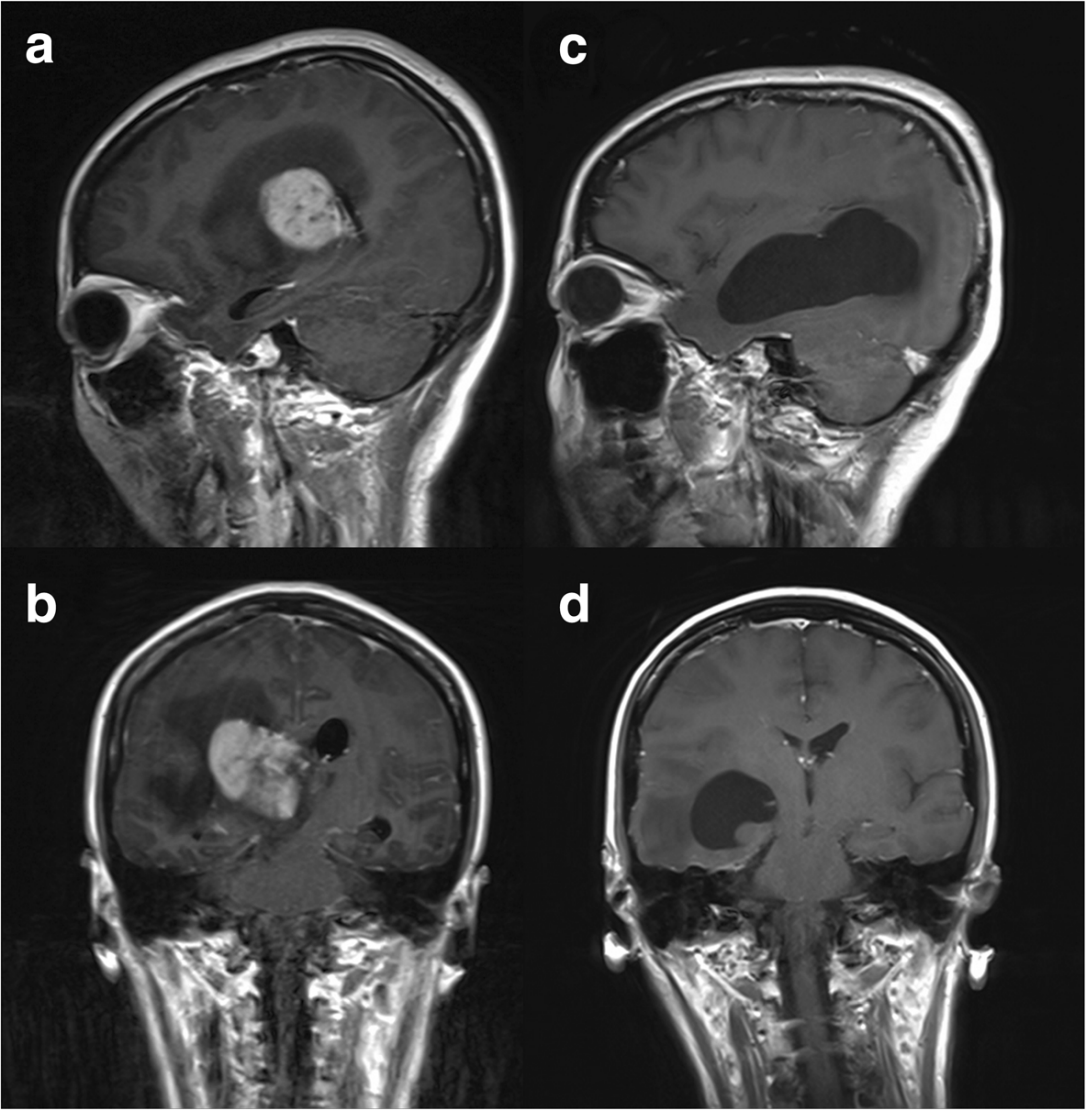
a, b MRI enhancement revealed: on the right side of the thalamus a block of long T2 long T1 signal can be seen,the boundary is clear, the range is 44 × 40 × 34 mm. Enhanced scanning showed inhomogeneous enhancement. Massive edema can be seen around the lesion. The third ventricle was compressed,bilateral ventricles dilated. Midline shift left slightly. c, d MRI enhancement after surgery and 5 cycles of R-MAD chemotherapy showed: right side of the occipital skull and soft tissue post operation change. The right lateral ventricles triangle and the temporal angle were irregular enlarged, the ventricular wall was less structured
